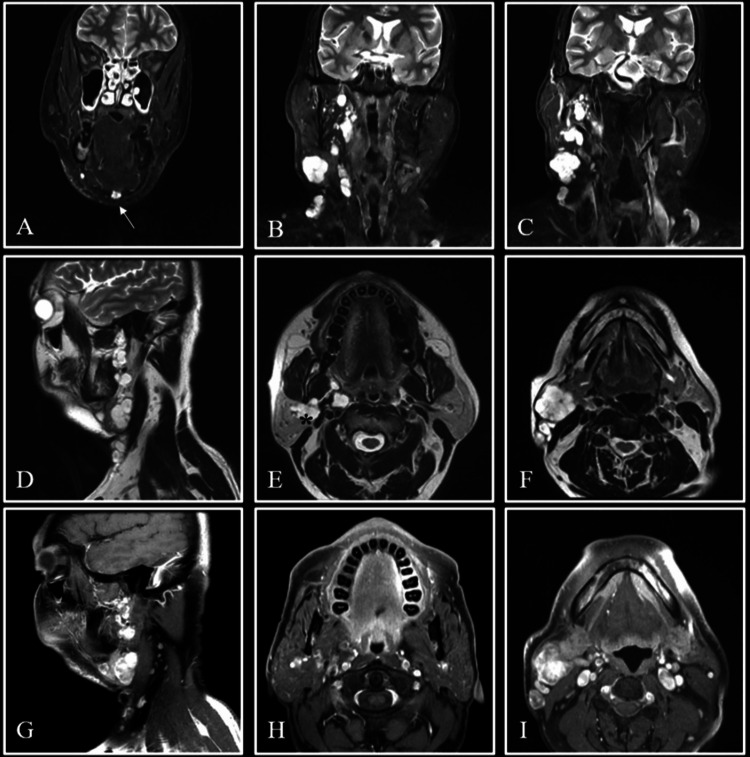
Imaging findings. Coronal STIR images (A, B, and C) show hyperintense lobulated lesions spanning the entire right side of the neck, extending from the base of the skull to the submandibular region inferiorly, and in the submental region (arrow). Sagittal (D) and axial (E and F) T2W images show multiple hyperintense lesions of similar morphology, involving the right side of the neck, the deep lobe of the parotid (asterisk), and the right submandibular gland. Post-contrast T1W images (G, H, and I) demonstrate enhancement within these lesions. STIR: short tau inversion recovery; T2W: T2-weighted; T1W: T1-weighted
